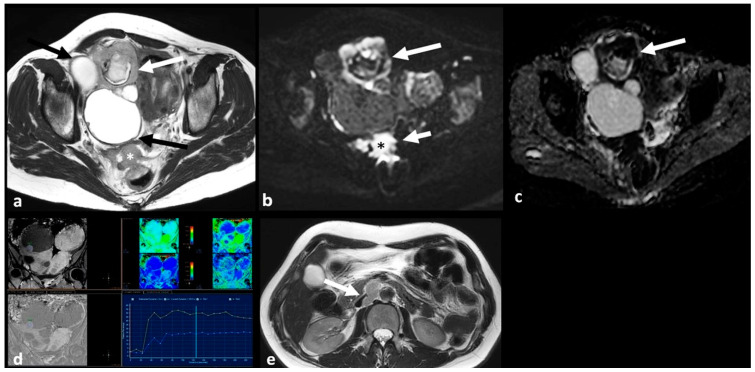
High grade serous cystadenocarcinoma with peritoneal and lymph node metastases. Axial T2 weighted image (a) shows a multiloculated right ovarian cystic mass (black arrows) with a large solid component (white arrow). The solid portion (white arrow) demonstrates high signal on axial 1200 b value DWI (b) and low signal on ADC map (c). DCE image analysis (d) demonstrates an intermediate-risk time intensity curve of the solid tissue (TIC type 2, orange line: myometrium, blue line: solid tissue). Axial T2 weighted image of the upper abdomen of the same patient (e) shows right paraaortic lymphadenopathy (white arrow). Shown also is a large peritoneal implant in the pelvis (asterisk in (a); asterisk and short arrow in (b)).
